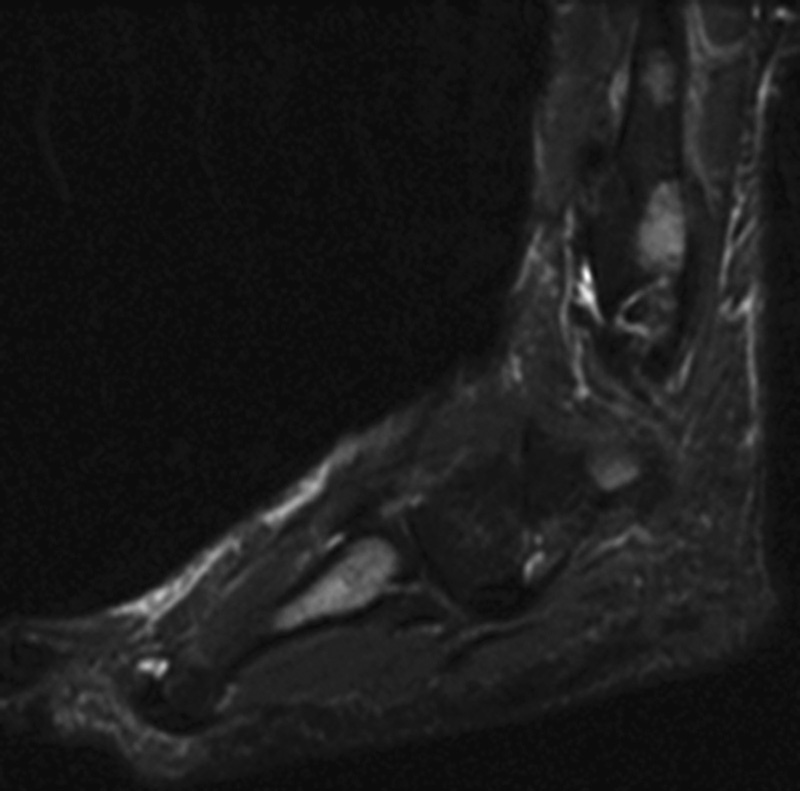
Hyperintense lesions in fibula and fourth metatarsal bone (T2-weighted magnetic resonance imaging section).
